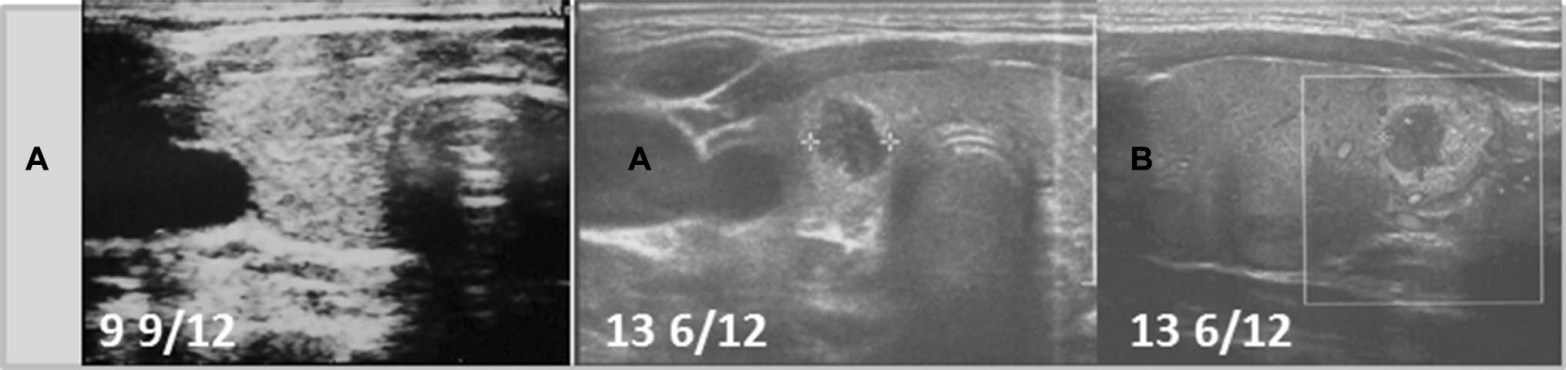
Patient 4. Transversal (a) and longitudinal (b) scans of the normoechogenic thyroid gland revealed the formation of the nodule 4 years since referral and 3 9/12 months since last ultrasound assessment. The nodule was surrounded by a hyperechogenic border. During follow-up, the echogenicity of the thyroid gland increased
